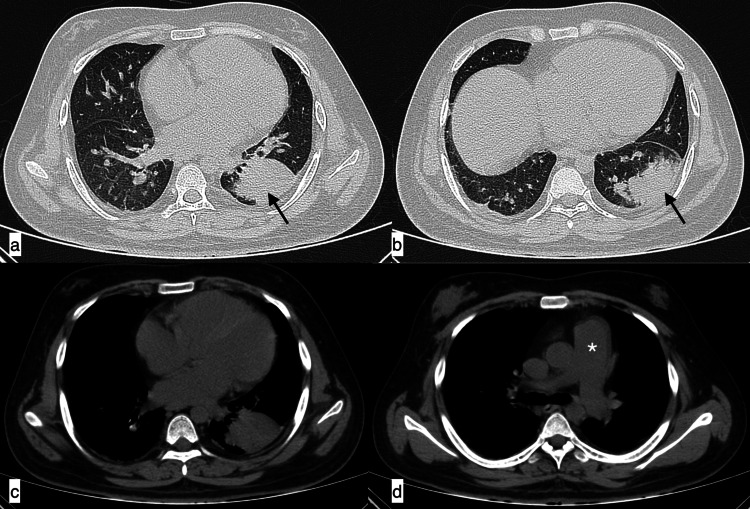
Infective aetiology A 27-year-old male patient with SLE presented with fever, shortness of breath, and cough of seven-day duration. Axial HRCT chest images in the lung window (a, b) revealed a large area of consolidation in the left lower lobe with minimal synpneumonic left pleural effusion (black arrows), suggestive of infective aetiology. Additionally, in the mediastinal window, cardiomegaly (c) and an enlarged pulmonary artery (d, asterisk) were observed. SLE: systemic lupus erythematosus; HRCT: high-resolution CT
